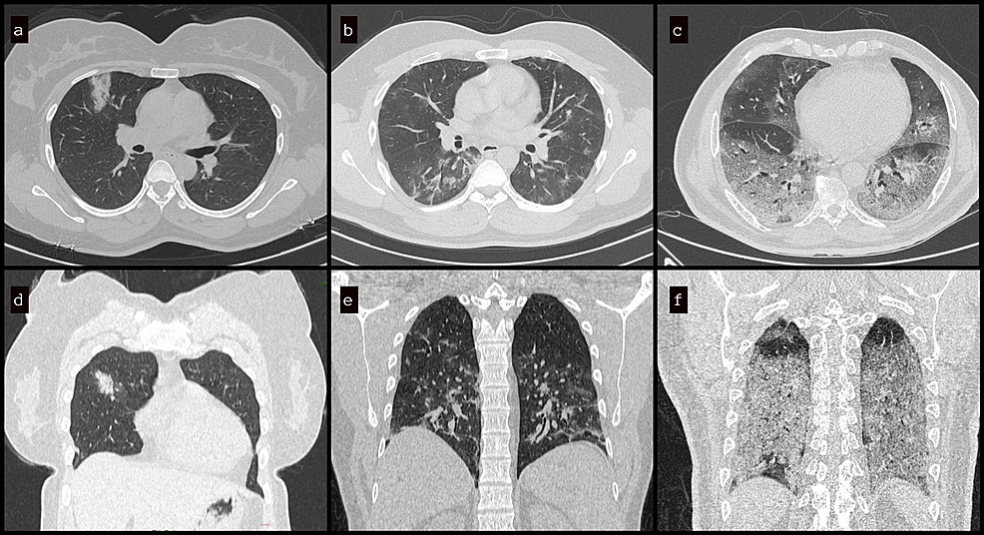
HRCT lung showing the extent of lung involvement in mild (a, d), moderate (b, e), and severe (c, f) disease based on CT scoring system HRCT: high-resolution computed tomography
